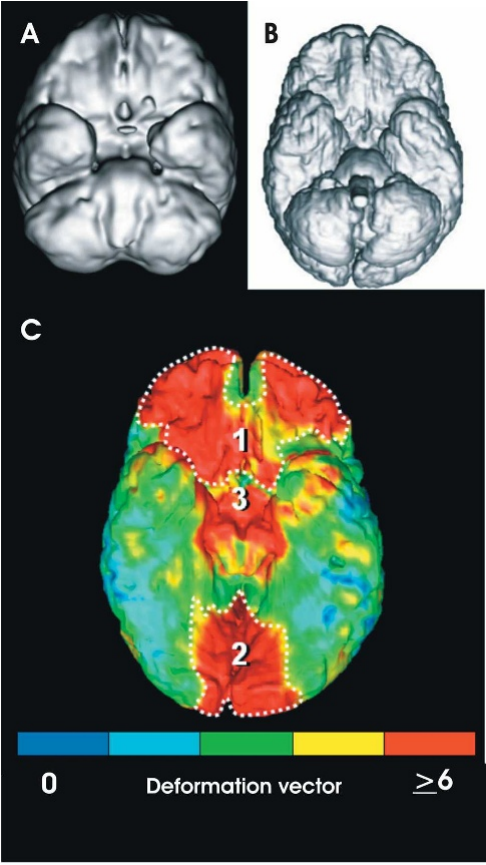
Magnetic Resonance Imaging of Brains Three-dimensional reconstruction of a reference bonobo (pygmy chimpanzee) brain (A) and a reference human brain (B) after magnetic resonance imaging and normalisation of absolute brain sizes. The virtual bonobo brain has been transformed into the virtual human brain using an elastic deformation algorithm. The local deformation vectors are colour-coded and projected onto the virtual human brain (C). The most dramatic changes in brain shape occur in (1) the ventro-orbital prefrontal cortex, (2) the ventral stream of the visual cortex, and (3) the hypothalamic neuroendocrine region. (Image: Karl Zilles, Hartmut Mohlberg, and Peter Pieperhoff, Research Center Jülich)
